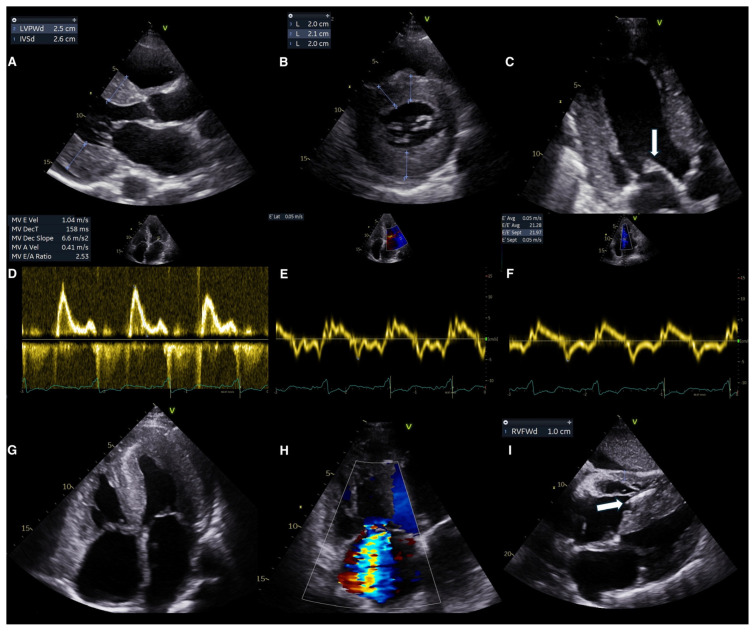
Conventional echocardiographic red flags in ATTR-CM. (A,B) Concentric hypertrophy of the left ventricle demonstrated at parasternal long axis view (A) and short axis view (B). (C) Thickened mitral valve leaflets (arrow). (D–F) Grade III diastolic dysfunction demonstrated by (D) Transmitral flow (E) tissue doppler of the lateral wall and (F) tissue doppler of the intraventricular septum. (G) Four chamber view demonstrating biatrial enlargement, interatrial septum thickening, right ventricular free wall hypertrophy and pericardial effusion. (H) Color doppler imaging demonstrating the significant tricuspid regurgitation. (I) Subcostal view demonstrating hypertrophy of the right ventricle and the thickened interatrial and interventricular septum, visible pacemaker lead in the right ventricle (arrow). ATTR-CM: transthyretin amyloid cardiomyopathy, IVSd: interventricular septum diameter, LVPWd: left ventricular posterior wall diameter, MV: mitral valve, MV DecT: mitral valve deceleration time, and RVFWd: right ventricular free wall diameter.
